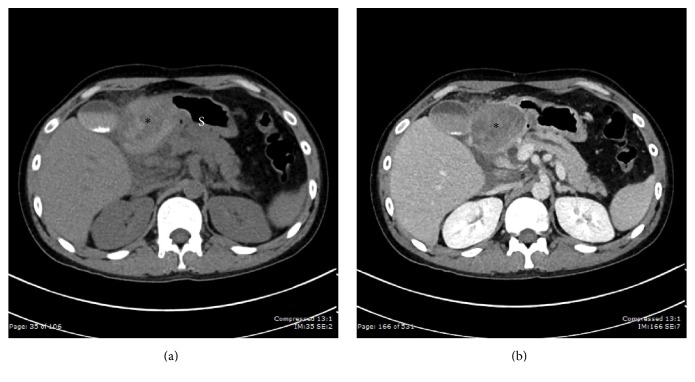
(a) Noncontrast CT scan shows a heterogeneously high-attenuation mass (*∗*) along the course of 1st, 2nd, and 3rd part of duodenum, which is compatible with duodenal hematoma. Note the surrounding fat stranding. S = stomach. (b) Contrast-enhanced CT shows the lack of enhancement within the mass (asterisk). Note the displacement of gas in gastric lumen secondary to mass.
